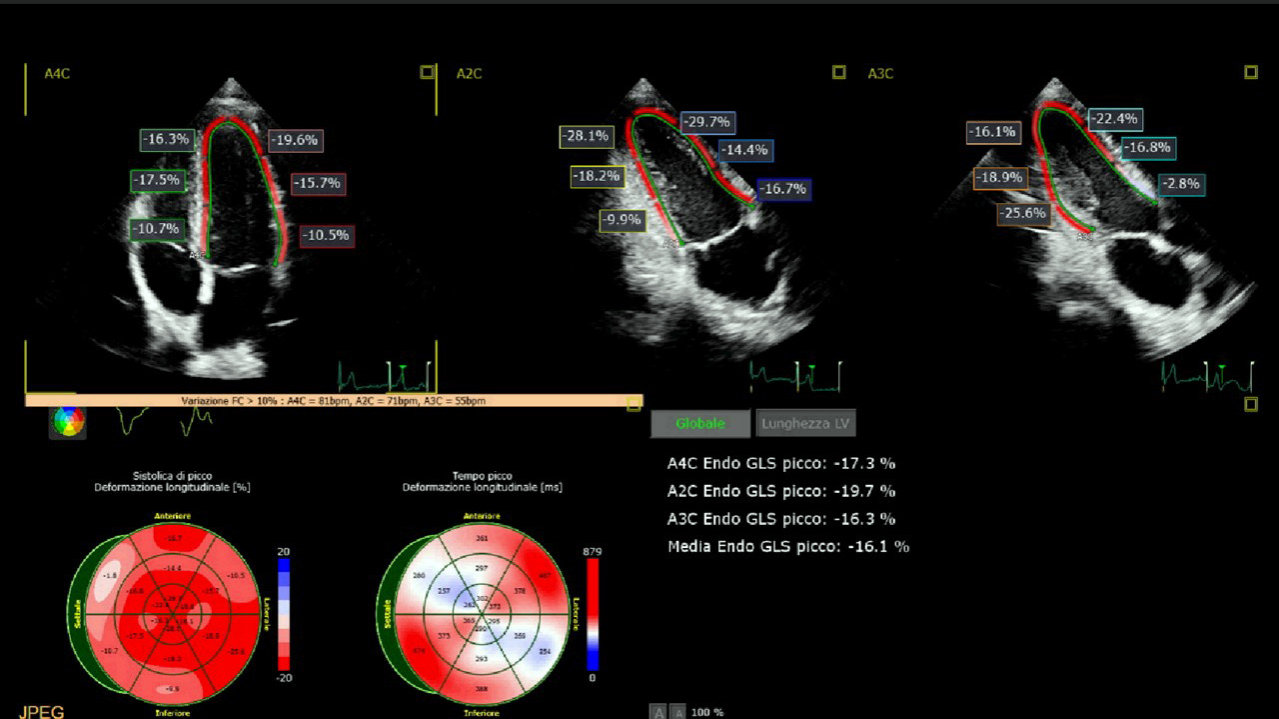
Echocardiographic analysis by speckle tracking: a global longitudinal strain (GLS) of −16.1% is seen.
